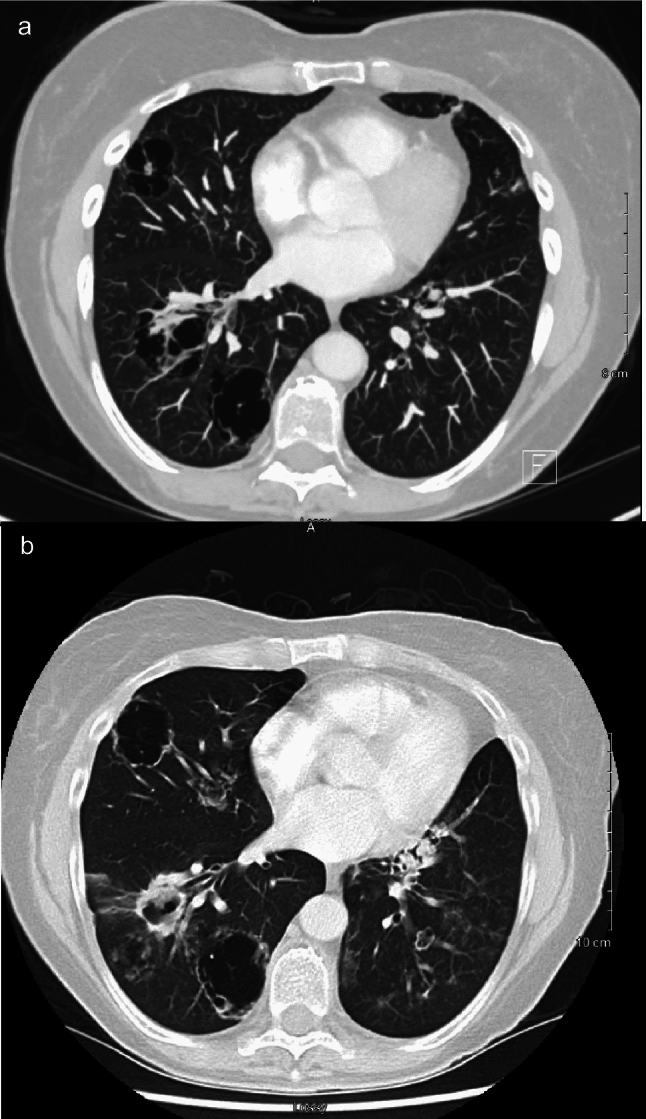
Computed tomography scan of a 58-year-old patient’s chest on initial presentation (a) and three months later (b). The scan on initial presentation demonstrates cystic masses in the lungs. The scan three months later depicts metastatic disease with ongoing superimposed invasive aspergillosis.
